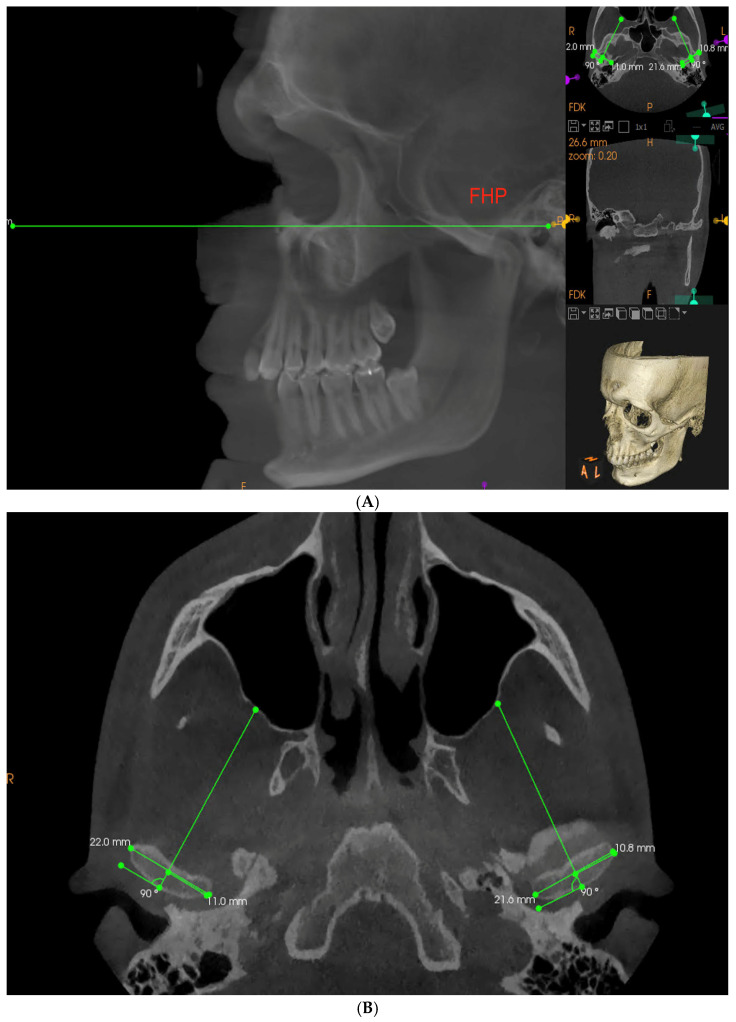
(A,B) Establishing a Frankfort reference plane and a proper CBCT layer to obtain reproducible measurements for all the patients according to anatomical landmarks (FHP—Frankfort horizontal plane).
